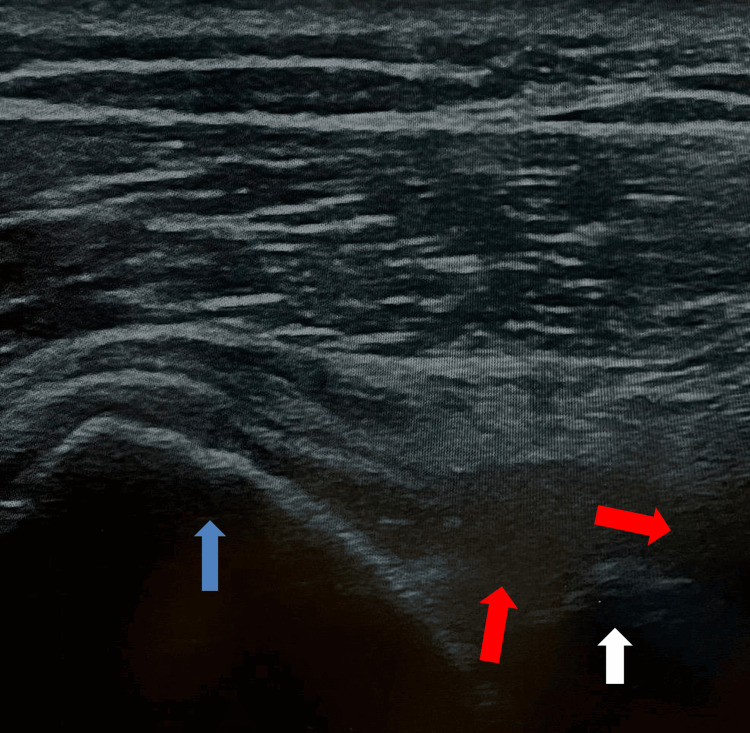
Ultrasound findings with joint effusion and a suspected paralabral cyst projecting into the spinoglenoid notch Blue arrow (humeral head); red arrows (joint effusion and paralabral cyst with extension to the spinoglenoid notch); white arrow (scapula).
